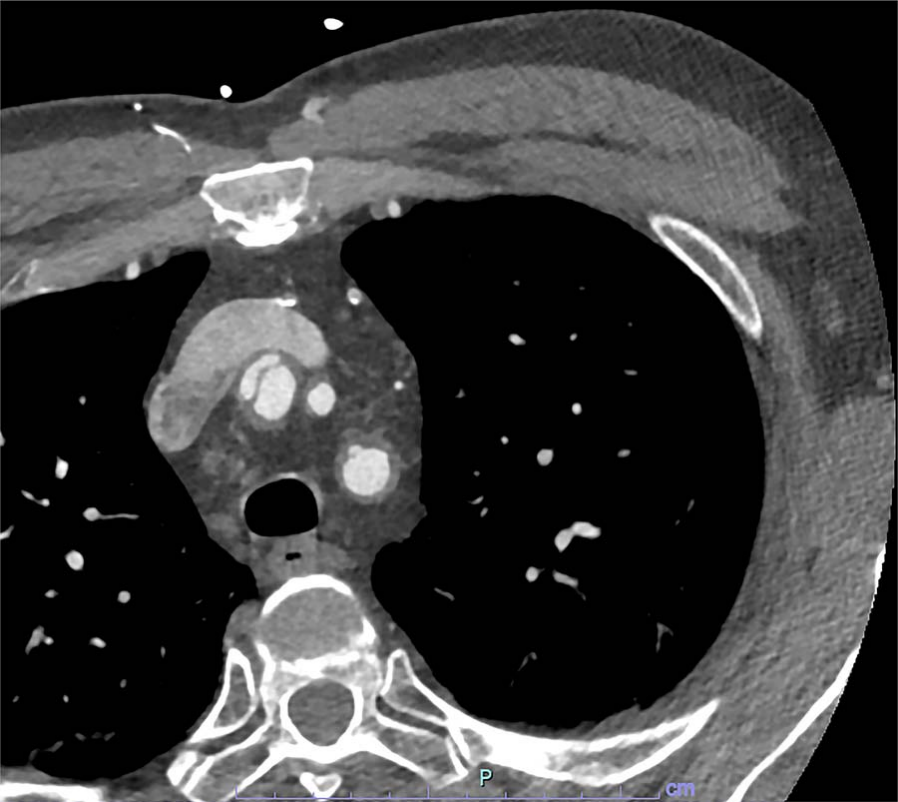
Axial slice from CT aortogram in greater detail of the dissection flap.
